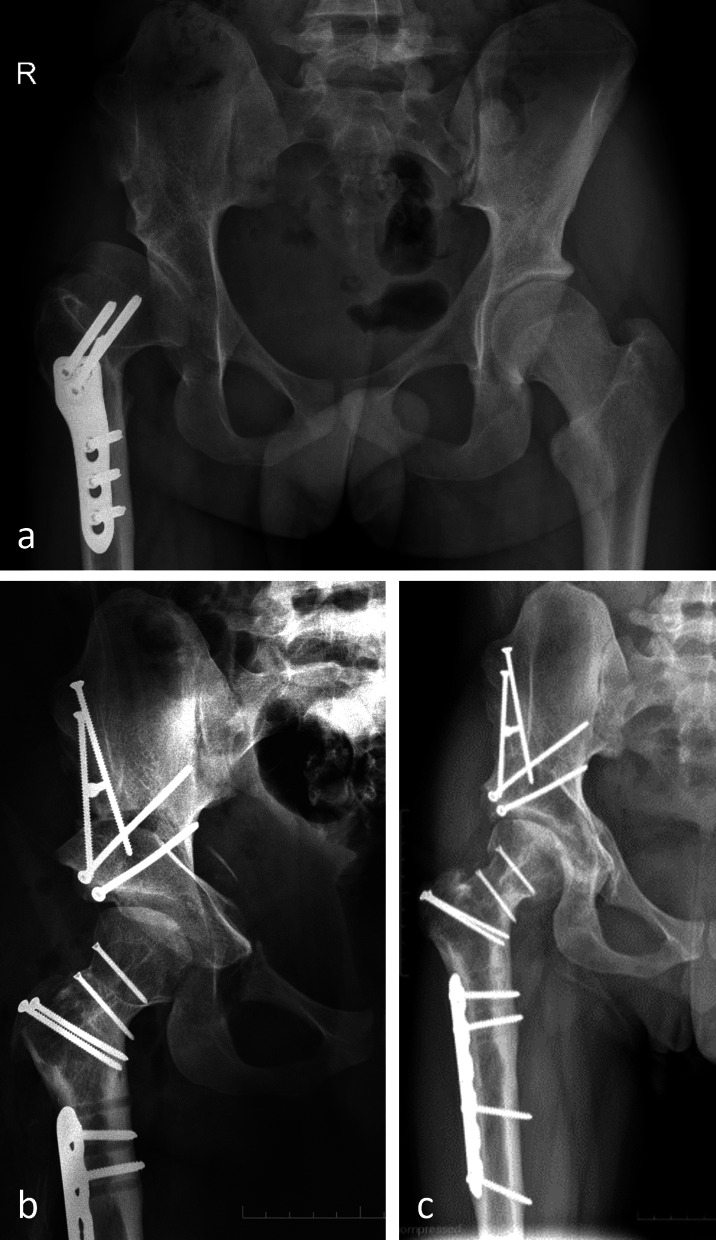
Severe deformity (courtesy Prof. P. Rego, Hospital da Luz, Lisbon, Portugal) of the right hip in a 16-year-old male after open reduction at the age of 1.5 years, followed by avascular necrosis. a Varus osteotomy at the age of 14 years, which did not improve limping and pain. b Postoperative radiography after complex surgery with relative lengthening of the neck, intracapital osteotomy to reduce the size of the head, and subtrochanteric derotation osteotomy followed by PAO. c The 4-year follow-up result with good and pain-free function
